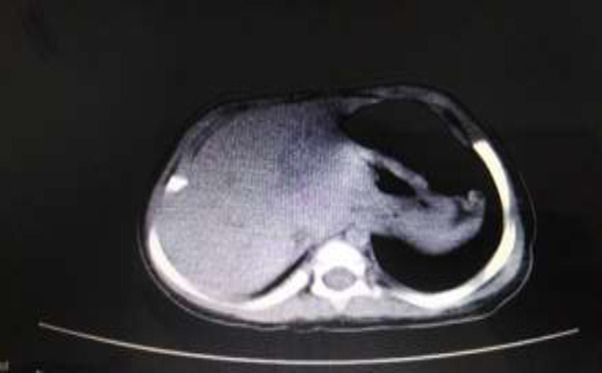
Abdominopelvic CT scan after paracentesis in the previous admission to another hospital
